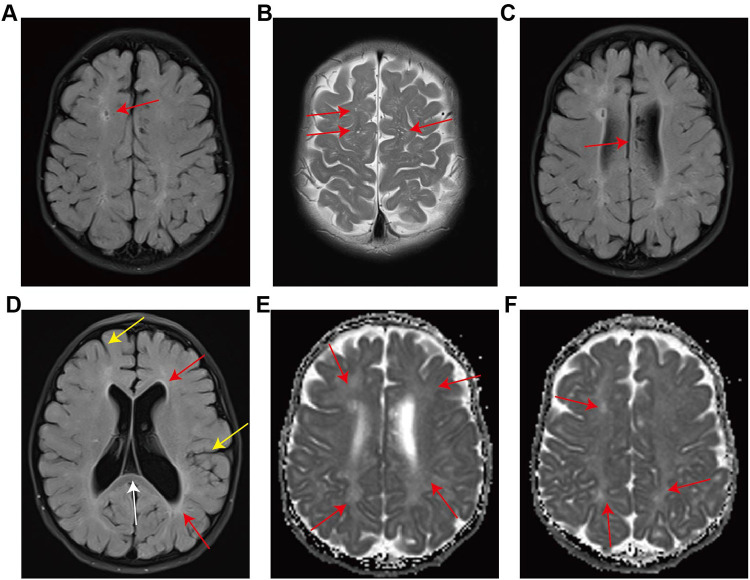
Cranial MRI. (A,C,D) Flair sequence image; (B) T2WI image; (E,F) Apparent diffusion coefficient sequence image.
